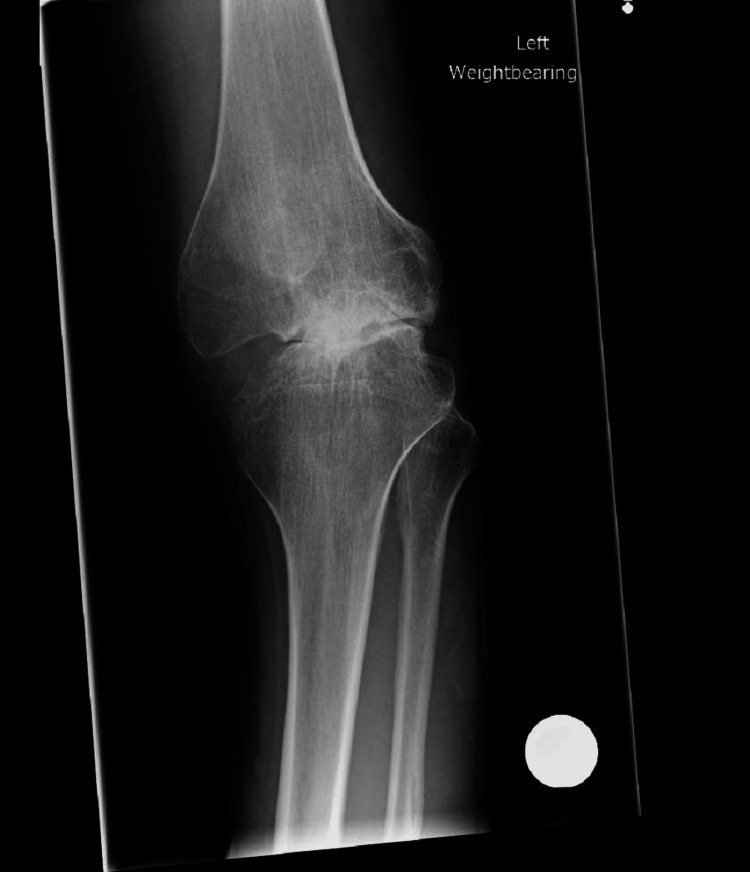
Preoperative anteroposterior X-ray of the left knee showing varus deformity and caudal sloping of the medial tibial plateau.
